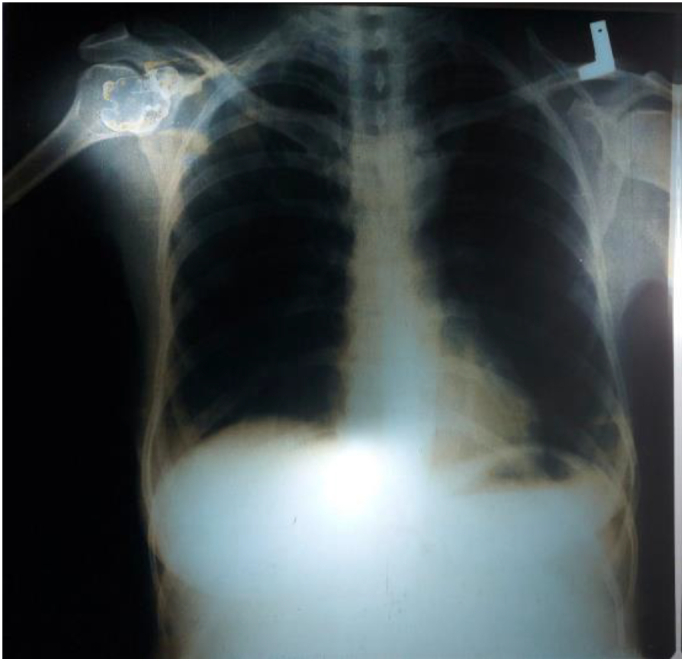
Showing, Normal chest radiograph.
